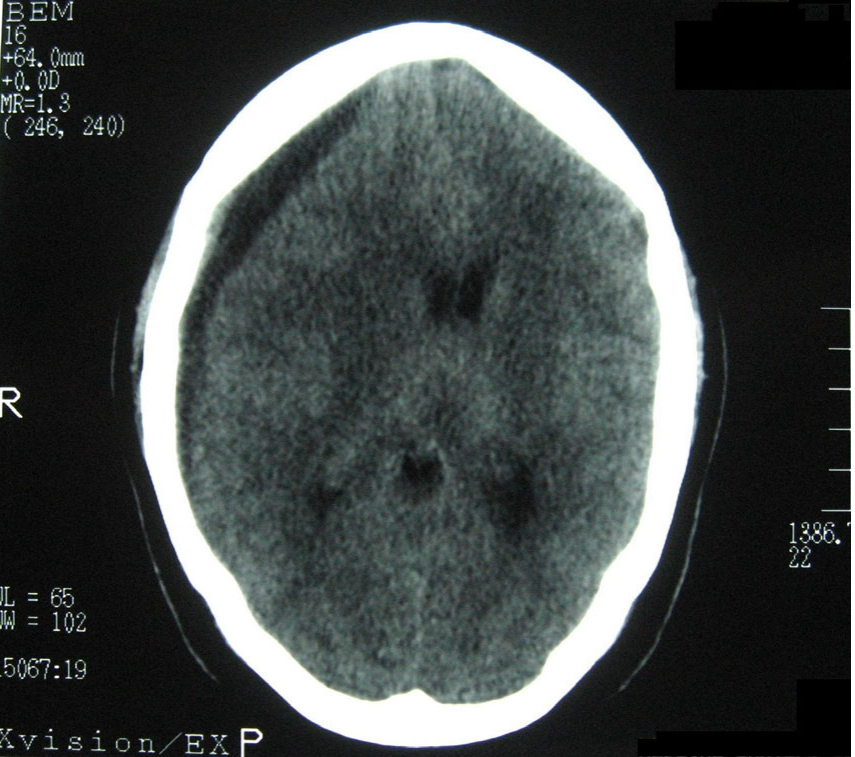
Noncontrast brain computed tomography showing a crescent-like hypodense area in the right fronto-parietal region with a tiny hyperdense rim in the lateral border of the lesion.
